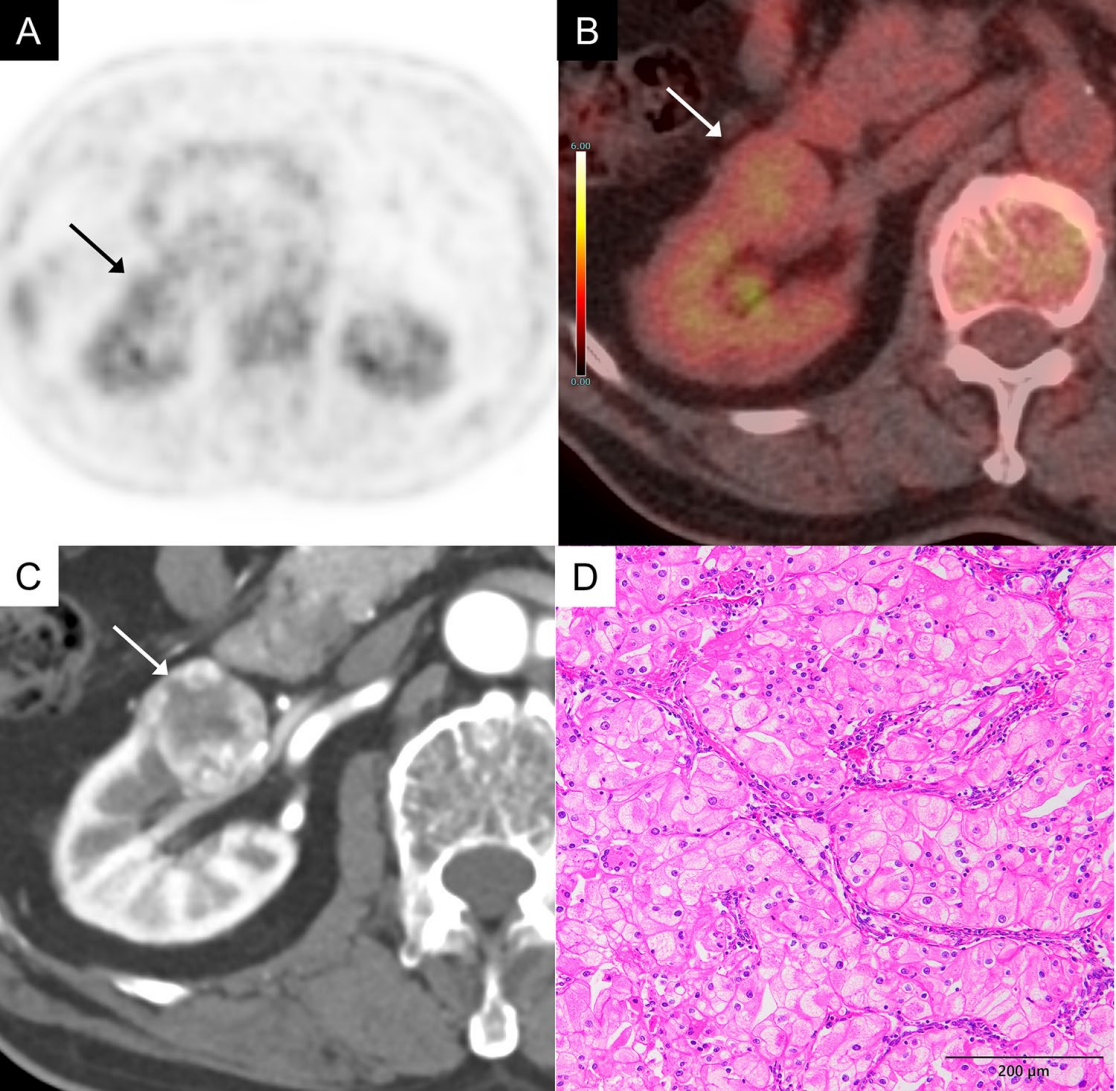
A 79-year-old man with a clear cell renal cell carcinoma (WHO/ISUP grade 3) of the right kidney. A Axial FDG-PET image (gray scale) shows mild FDG uptake in a mass of the right kidney (arrow). B Axial FDG-PET/CT image shows mild FDG uptake in a mass of the right kidney (arrow), showing an SUVmax 2.9, while normal renal cortex shows an SUVmax 2.3. C Axial contrast-enhanced CT in the corticomedullary phase shows a heterogeneously intense enhancement in the mass (arrow). D The tumor cells have distinct, eosinophilic nucleoli and clear cytoplasm. These nucleoli are visible at low magnification (× 100)
